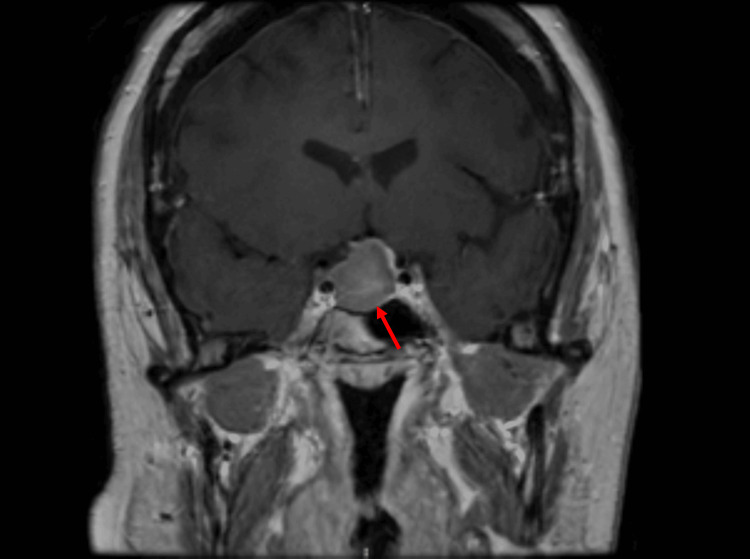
Contrast-enhanced coronal view of the MRI pituitary scan eight months after initial presentation. The appearance of the pituitary gland (red arrow) remained stable, with no change in size or morphology of the mass.
